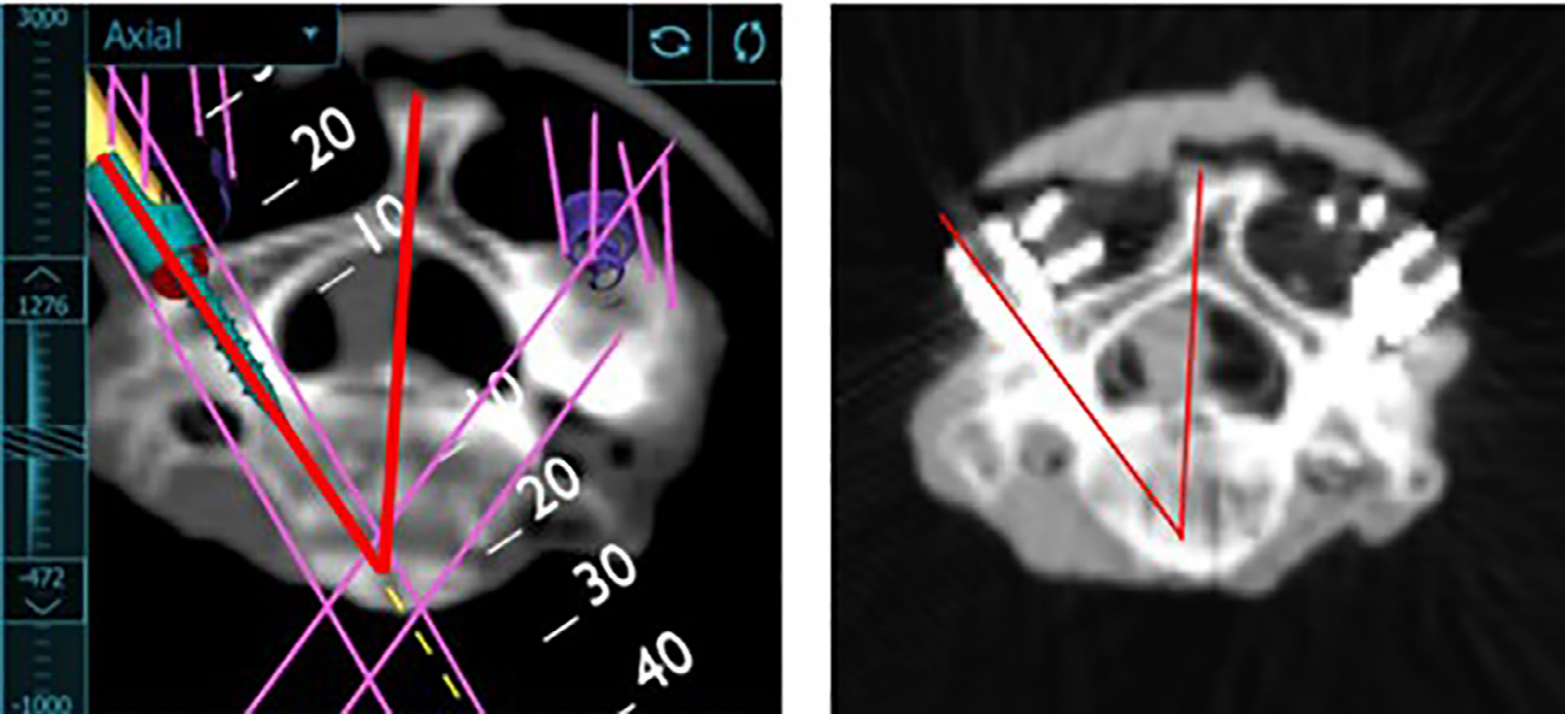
Axial image of final planned screw position from screen shot and axial CT scan image of final actual screw position from postoperative CT scan. Red lines indicate angles measured.
